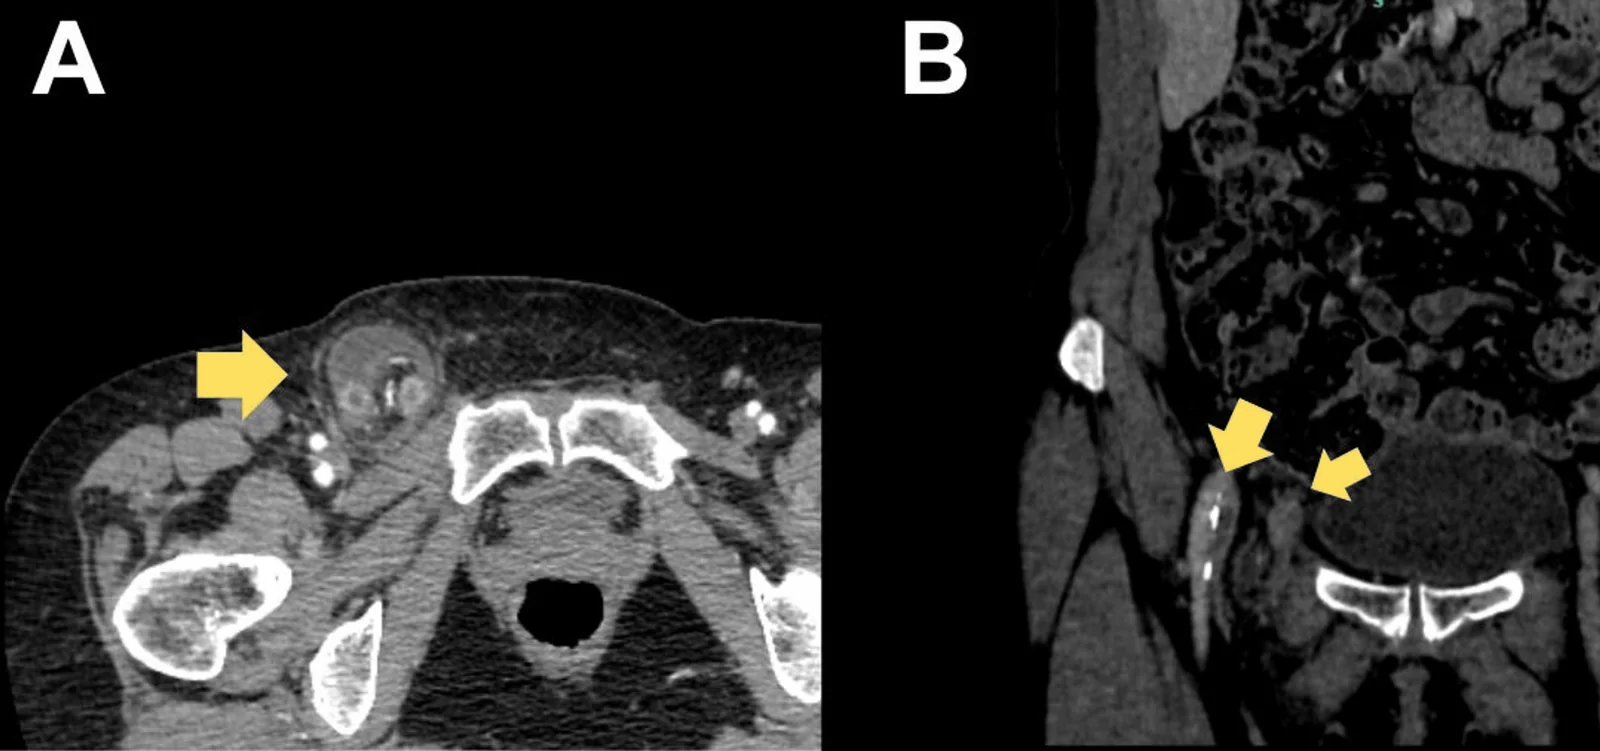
Computed tomography scans showing de Garengeot's hernia and deep vein thrombosis (A) Axial CT view demonstrating the right femoral hernia containing an inflamed vermiform appendix with parietal contrast enhancement and surrounding fat stranding; (B) Coronal CT view demonstrating an acute, extensive deep vein thrombosis (DVT) with a clear intraluminal filling defect involving the right external iliac and common femoral veins (lateral arrow), directly caused by mechanical extrinsic compression from the tense, adjacent hernia sac (medial arrow).
